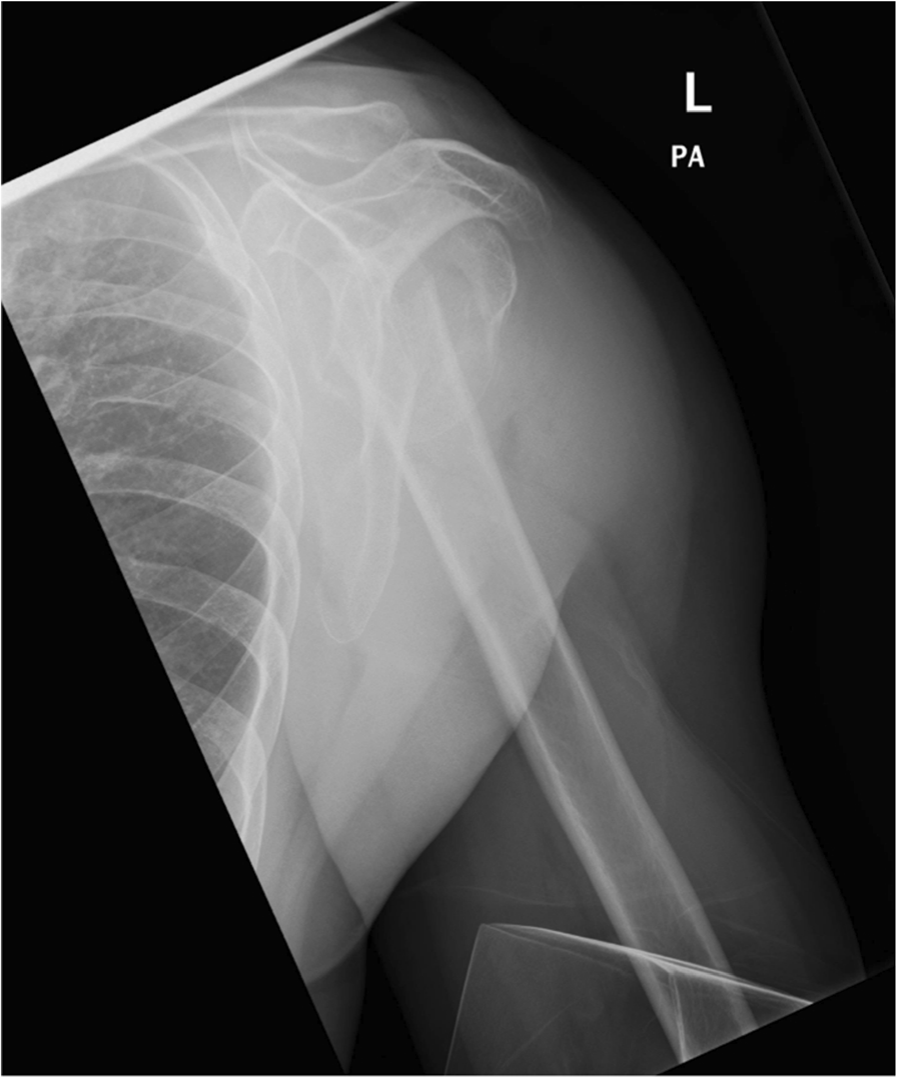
X-ray after trauma (Y-view).
